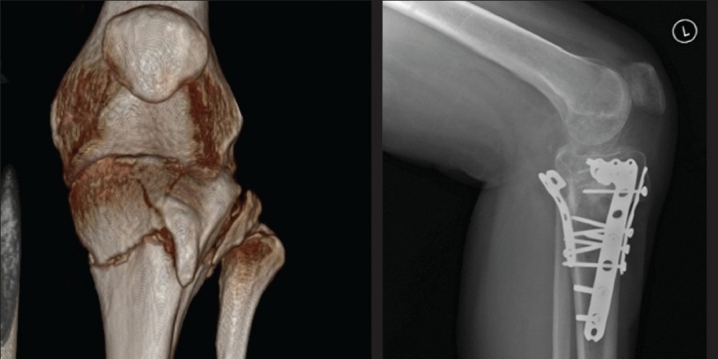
3D CT reconstruction showing tibial tuberosity fracture. X-ray (lateral view) of knee joint with proximal half of tibia showing sequence of stabilization of this fracture - posteromedial plate, tibial tuberosity fragment stabilized with anterior plate and lateral plate
